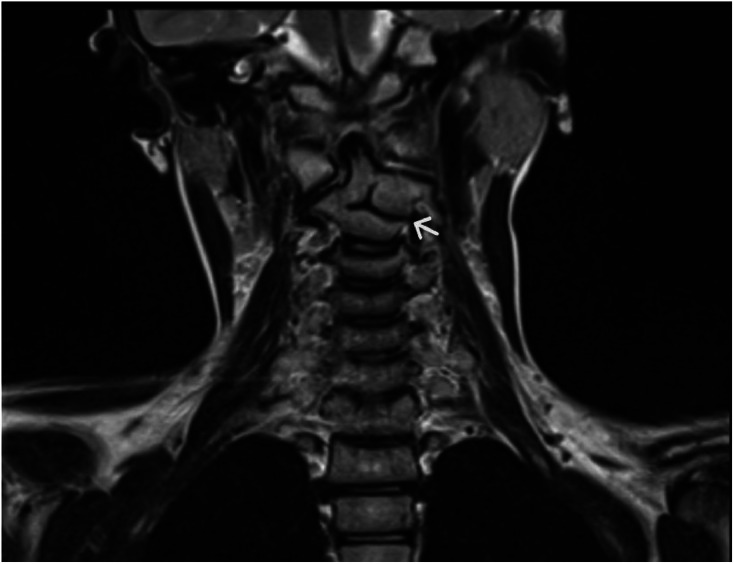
Coronal T2 magnetic resonance imaging (MRI) sequence confirming incomplete fusion of the C2 and C3 vertebral bodies, with deviation of the odontoid process to the right (white arrow).
